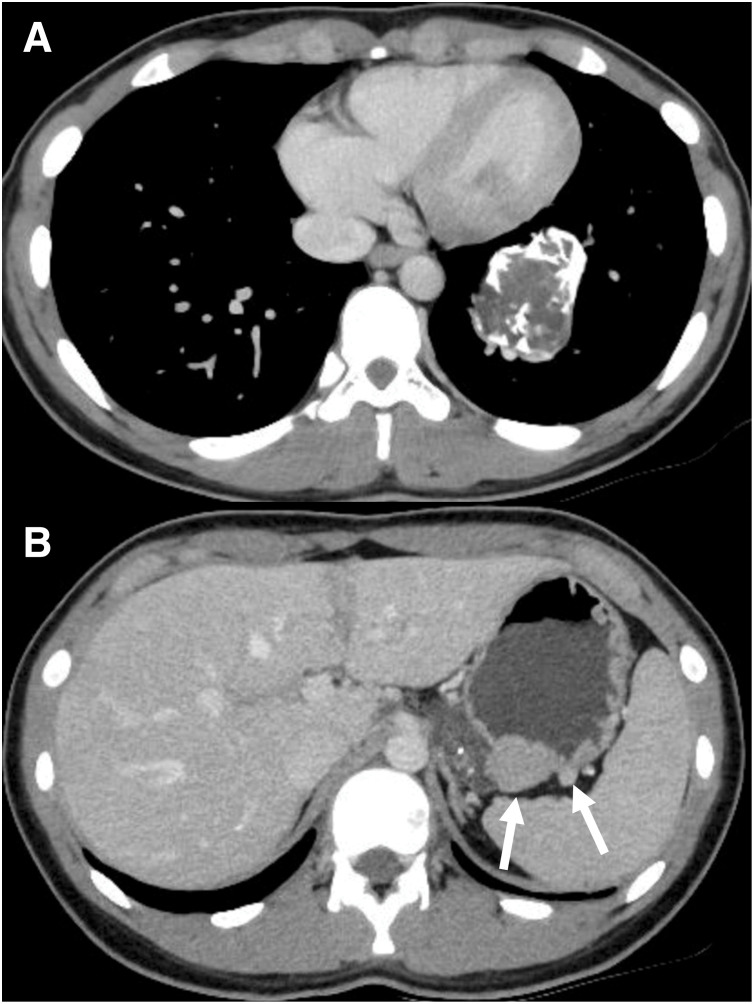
CT. (A) 55-mm pulmonary nodule with coarse calcification in the left lower lobe. (B) Gastric tumors in the upper body.
